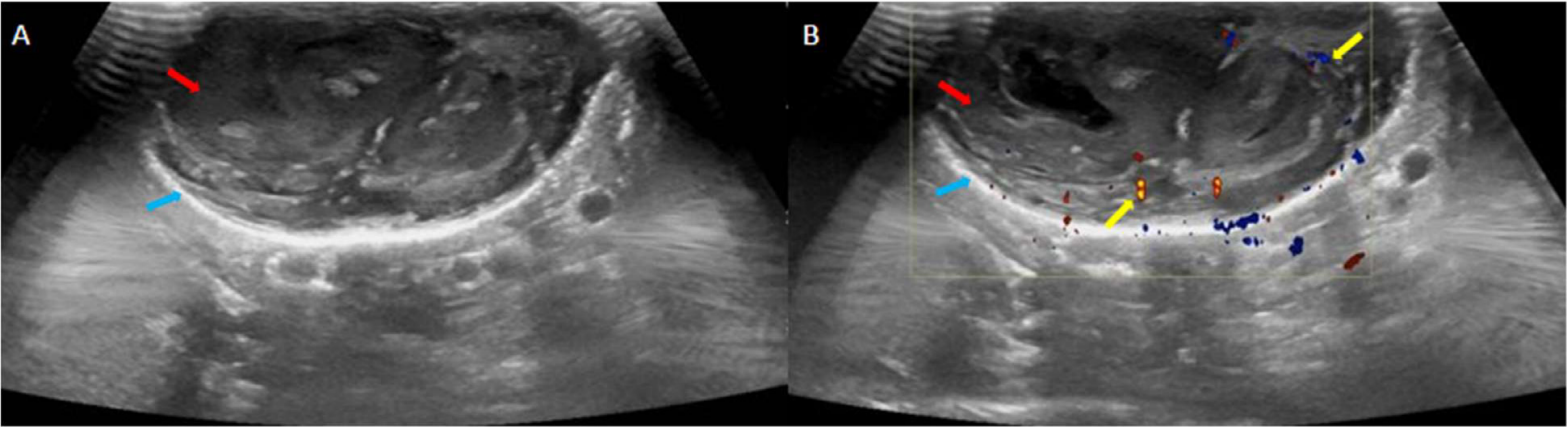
Ultrasound scrotum (A & B) showing a well-defined lesion of mixed echotexture in the scrotal wall (Red arrows), with an echogenic wall (blue arrows) and necrotic or turbid internal components. The solid-appearing components show areas of increased vascularity on the color Doppler (yellow arrows).
